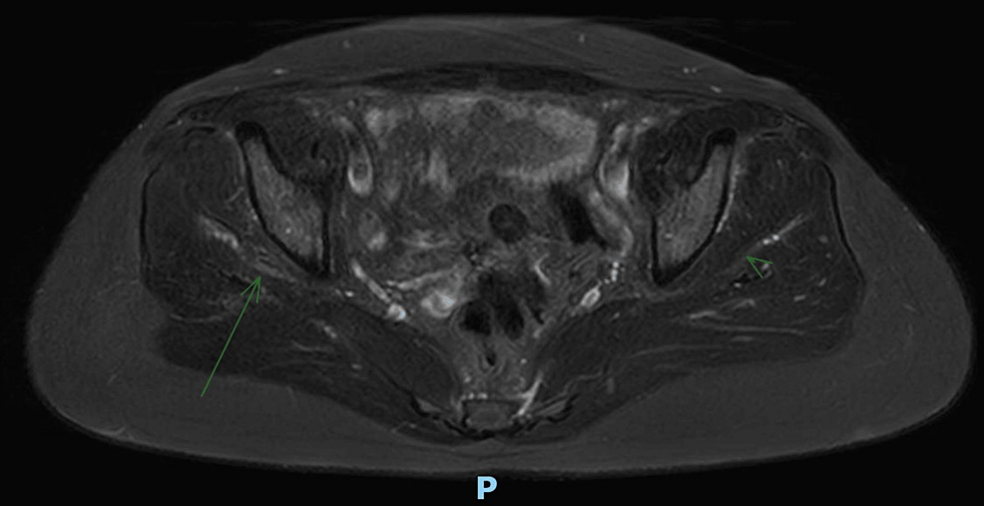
T2-Weighted MRI: Axial cut Ipsilateral hypertrophy of the gluteus medius (large arrow) and contralateral atrophy of the gluteus medius (small arrow).
